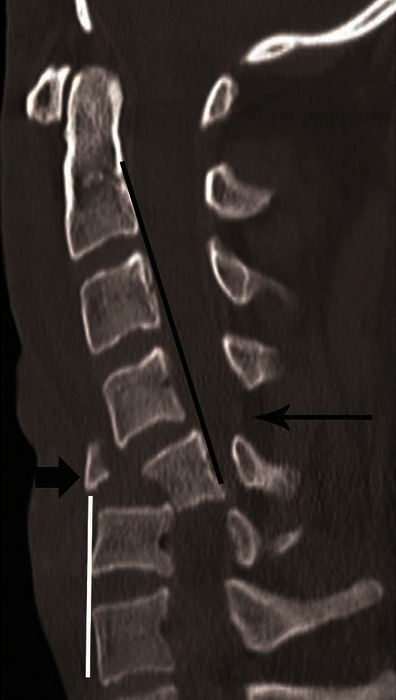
Flexion teardrop fracture. Sagittal CT image of cervical spine in a young patient following vehicular accident shows wedging of C5 vertebral body with an oblique coronal fracture of the anterior third of the vertebral body, dividing the C5 in to a smaller anterior triangular fragment (*short arrow*) and a large posterior segment. Retrolisthesis of the C5 vertebral body on C6 behind the fracture line and retropulsed C5 keeping alignment with the cranial C4 vertebral body—suggested by non-interrupted posterior vertebral line (*black line*), while triangular fragment keeping alignment with the caudal C6 vertebra—suggested by non-interrupted anterior spinal line (*white line*). Distraction of posterior column is suggested by widening of interspinous/interlaminar space at C4–C5 (*long arrow*). Facet joint subluxation was seen on the parasagittal images (not shown)
